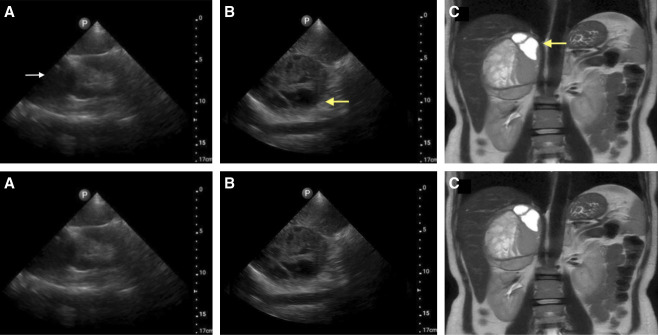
Imaging of adrenal mass. (A) Renal POCUS short axis view reveals a large hypoechoic mass abutting the right kidney (white arrow). (B) Renal POCUS long access view reveals the mass is well circumscribed, heterogeneous, and loculated (yellow arrow). (C) MRI redemonstrates the hemorrhagic right adrenal mass with loculated cysts containing blood-fluid levels (yellow arrow). MRI, magnetic resonance imaging.
